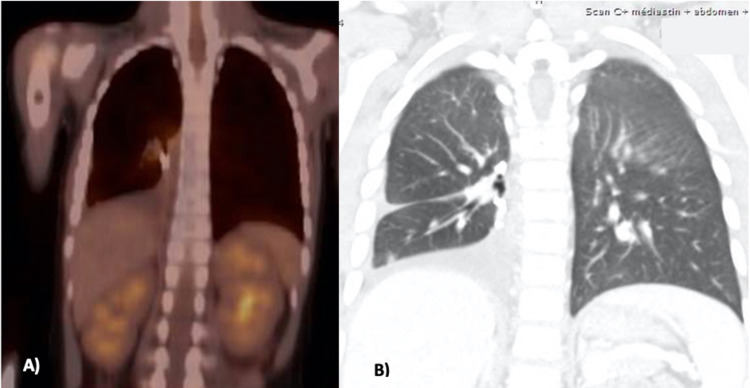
Imaging at the second relapse A) PET scan, B) CT scan
